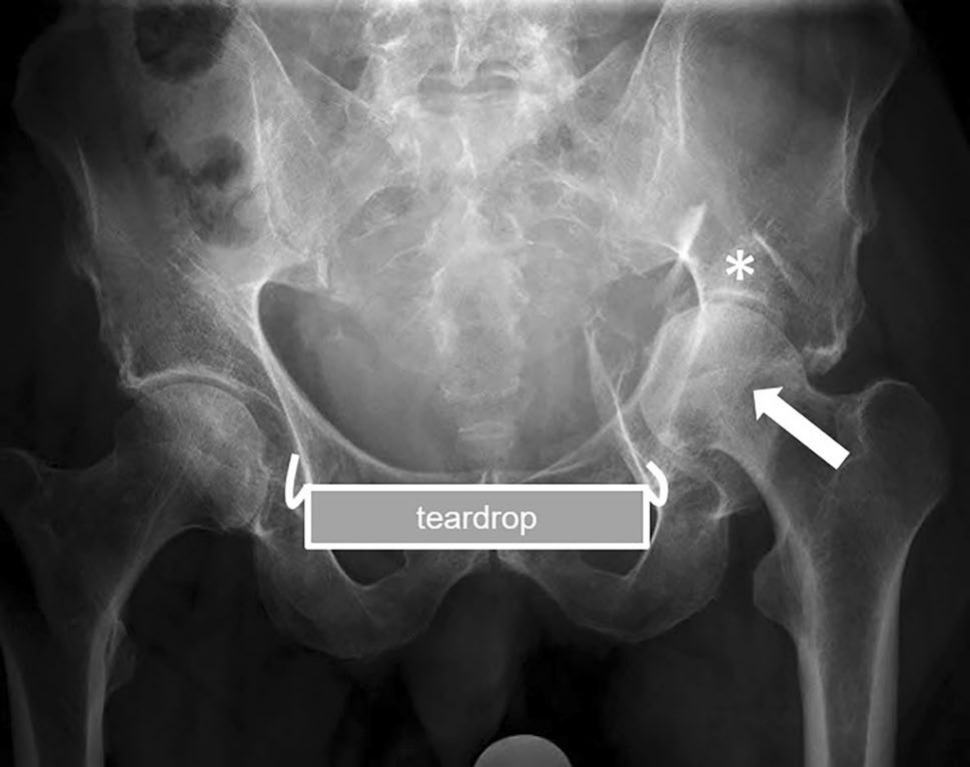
Preoperative anteroposterior radiograph of the pelvis showing an acetabular fracture (incomplete anterior column posterior hemitransverse) with break-out of the quadrilateral plate, impaction at the acetabular roof (asterisk) and medial subluxation of the femoral head in a 79-year-old male patient
